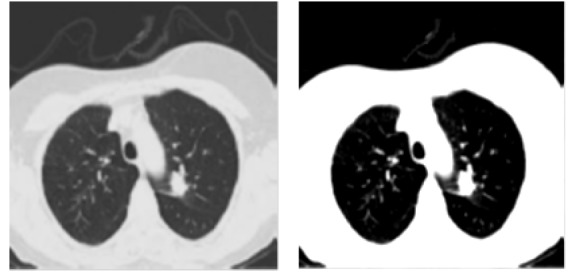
Cancerous and Binary CT Lung Image
